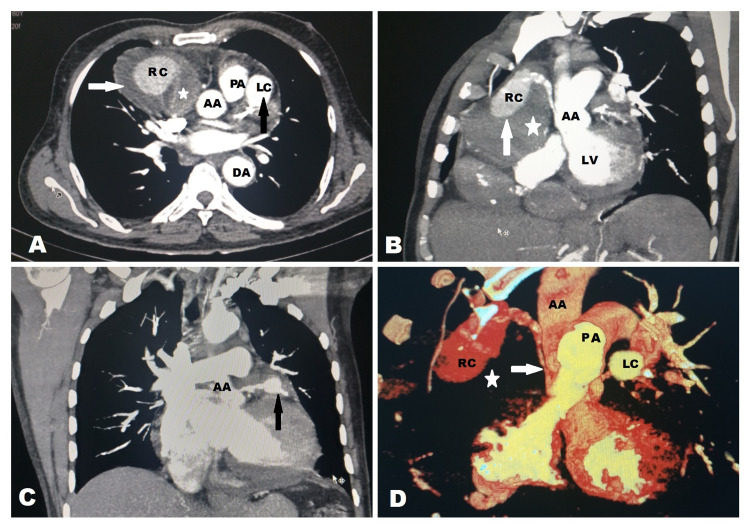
CECT of the thorax showing aneurysms arising from both right coronary artery and left anterior descending artery A: CECT in axial sections showing aneurysm (80 x 60 mm) (arrows) arising from the RCA (RC) with mural thrombus (star) and left LAD (30 x 30 mm) (LC) without mural thrombus (black arrow). Note the ascending aorta (AA), descending aorta (DA), and main pulmonary artery (PA). B: Maximum intensity projection (MIP) CECT in the oblique coronal plane showing the RCA arising from ascending aorta (AA) with aneurysm (RC) with mural thrombus (star). Note the ascending aorta (AA) and left ventricle (LV). C: MIP CECT in the coronal section showing the LAD arising from the ascending aorta (AA) with aneurysm (black arrow). D. Volumetric reconstructed CT scan showing the aneurysm from the RCA (RC) and LAD (LC). Note the mass effect on the proximal RCA (white arrow) at its origin from the ascending aorta (AA) due to aneurysm with mural thrombus component (white star) CECT: contrast-enhanced computed tomography: RCA: right coronary artery; LAD: left anterior descending
